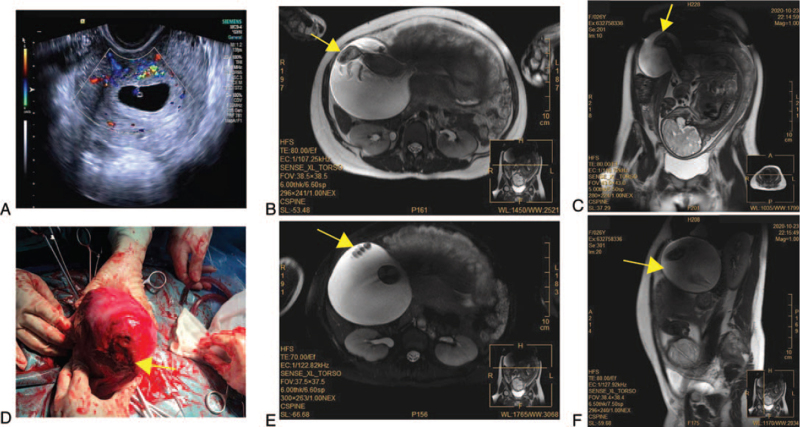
Ultrasound, magnetic resonance imaging, and surgical image of the patient. (A) Ultrasound demonstrated a gestational sac implanted in uterine cavity in the first trimester. (B-C and E-F) MRI demonstrated a dehiscence at the right aspect of the uterine horn with hernial sac containing amniotic fluid and foetal lower limbs protruding out of the peritoneal cavity. (D) The myometrium was completely ruptured at the primary surgical scar.
